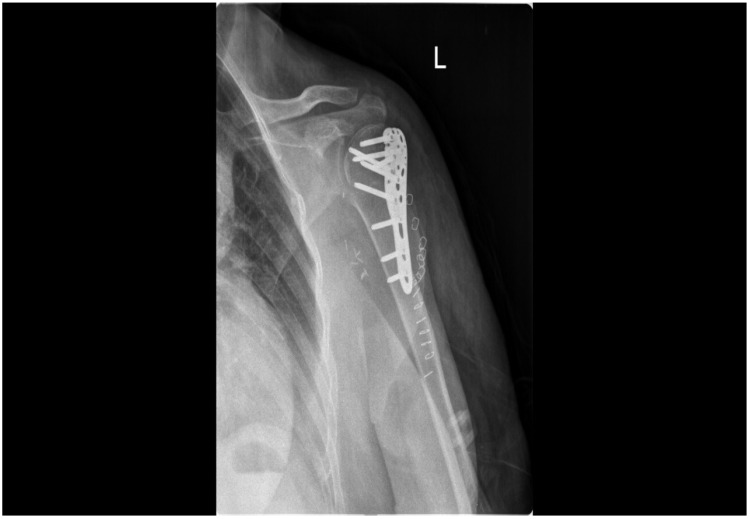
Post-op X-ray; Abbreviation: L—left.
